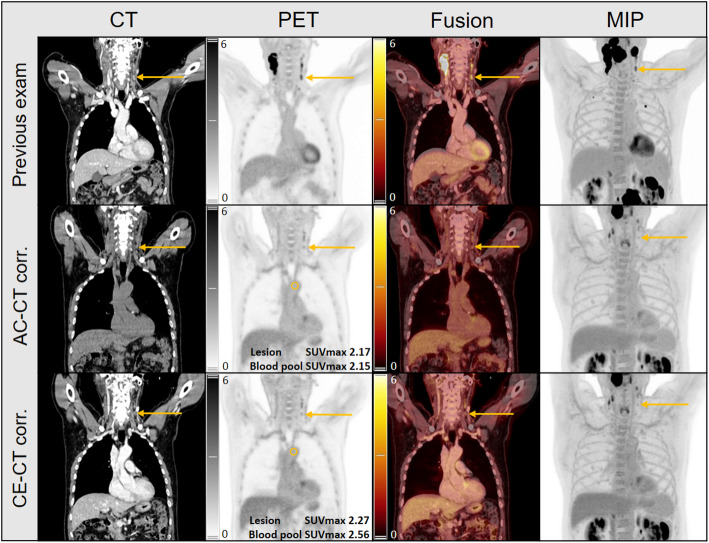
Higher mediastinal blood uptake with CE-CT PET reconstruction. Previous PET, restaging PET with AC-CT reconstruction and restaging PET with CE-CT reconstruction are shown. The marked lymphoma lesion (lymph node) showed an SUVmax higher than the blood pool when PET was reconstructed using CE-CT, whereas it was lower than the blood pool when reconstructed with AC-CT. An arrow indicates the lymph node, and the blood pool is outlined with a circle. PET window: SUV-bw 0–6; AC-CT window: center 40 HU, width 300 HU; CE-CT window (including CT of previous exam): center 45 HU, width 315 HU
